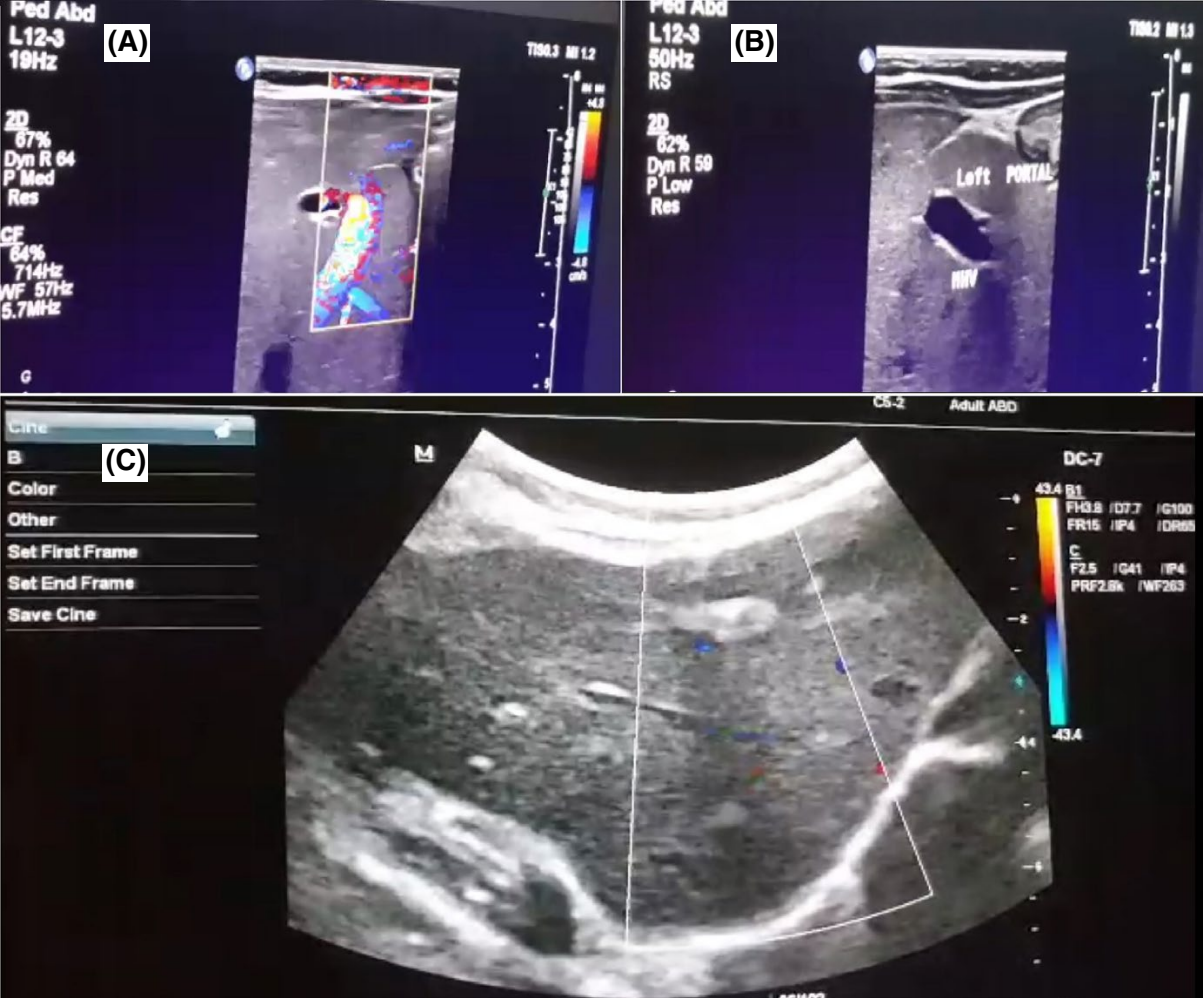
A, color Doppler mode ultrasonography before coil insertion; B, Gray‐ scale ultrasonography before coil insertion; C, Color Doppler mode ultrasonography after coil insertion
